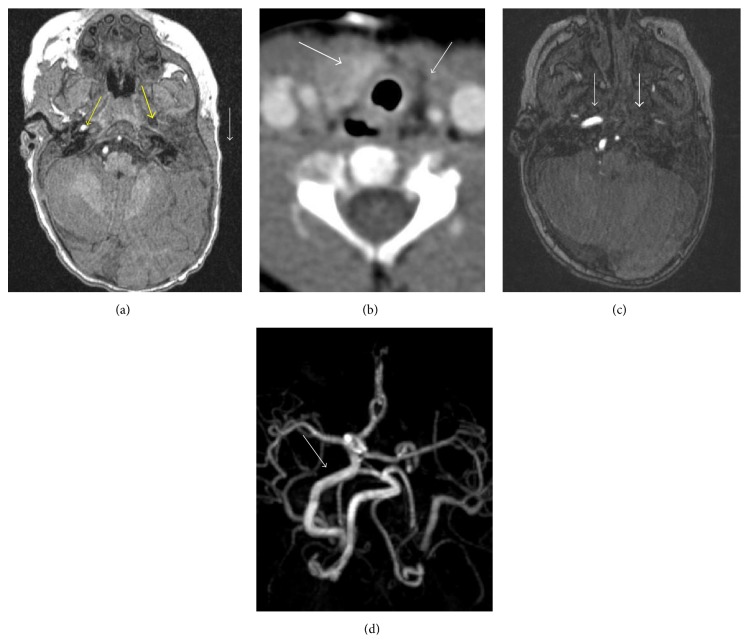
(a) Axial T1 weighted MR of the brain without contrast through the level of the external auditory canals demonstrates absence of the left external ear and external auditory canal (thin white arrow). There is also absence of the left internal carotid artery (thick yellow arrow). The right internal carotid artery is shown for comparison (thin yellow arrow). (b) Axial postcontrast CT of the neck at the level of the thyroid gland shows a normal right thyroid lobe (thick white arrow) with absence of the left thyroid lobe (thin white arrow). (c) Axial noncontrast time of flight MRA of the brain demonstrates a normal right internal carotid artery at its petrous segment (thin white arrow). There is absence of the left internal carotid artery (thick white arrow). (d) Three-dimensional reformation of the MRA of the brain demonstrates a normal right internal carotid artery (thin white arrow). The left internal carotid artery is absent.
